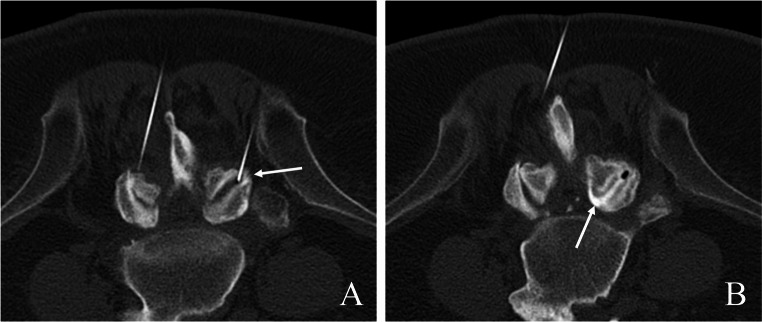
Typical example of simultaneous bilateral facet joint infiltration L4/L5 in a 56-year-old male patient, performed in prone position. (A) documentation of final needle position intraarticularly within the right facet joint L4/L5 (white arrow). (B) final CT-documentation for the right facet joint, proving proper intraarticular distribution of the drug cocktail (local anesthetics and cortical steroids mixed with iodinated contrast medium, white arrow)
